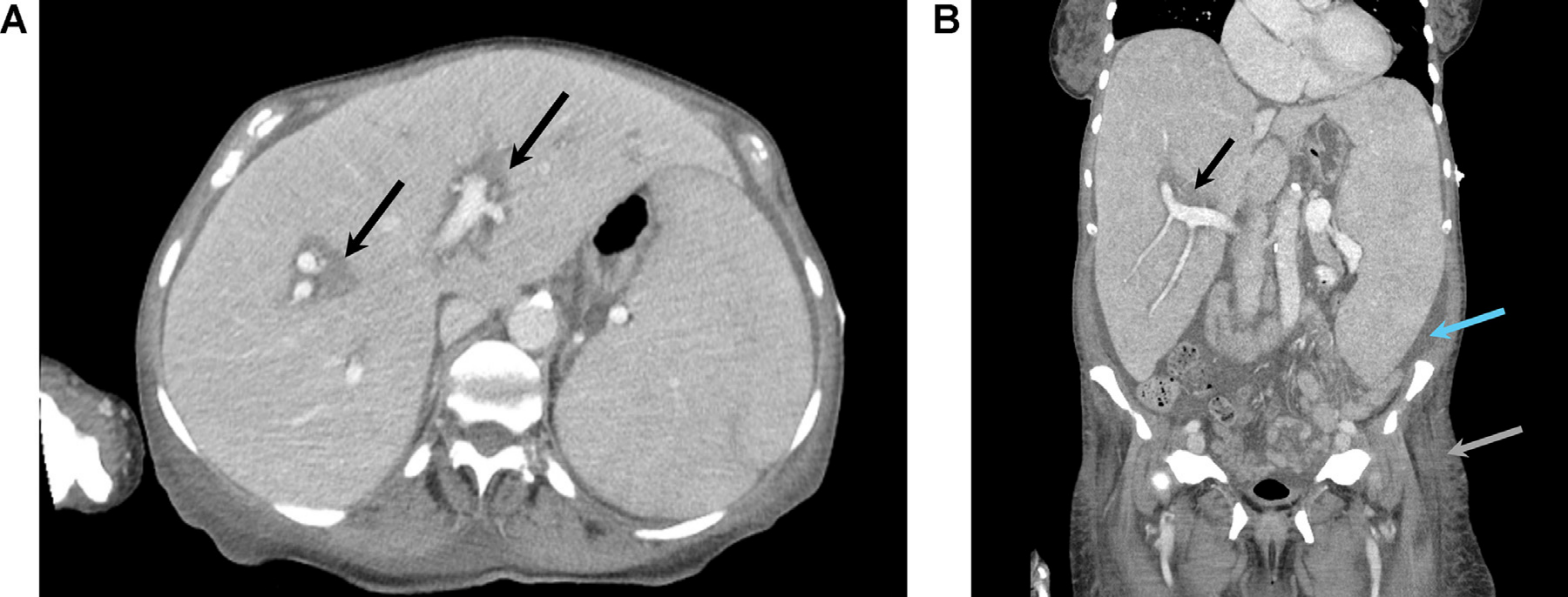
(A) Axial CT of the abdomen with IV contrast shows hepatosplenomegaly. There is periportal low attenuation, likely representing periportal edema (black arrows). (B) Coronal CT of the abdomen with IV contrast shows hepatosplenomegaly. There is periportal edema (black arrow), ascites (blue arrow), and anasarca (gray arrow).
